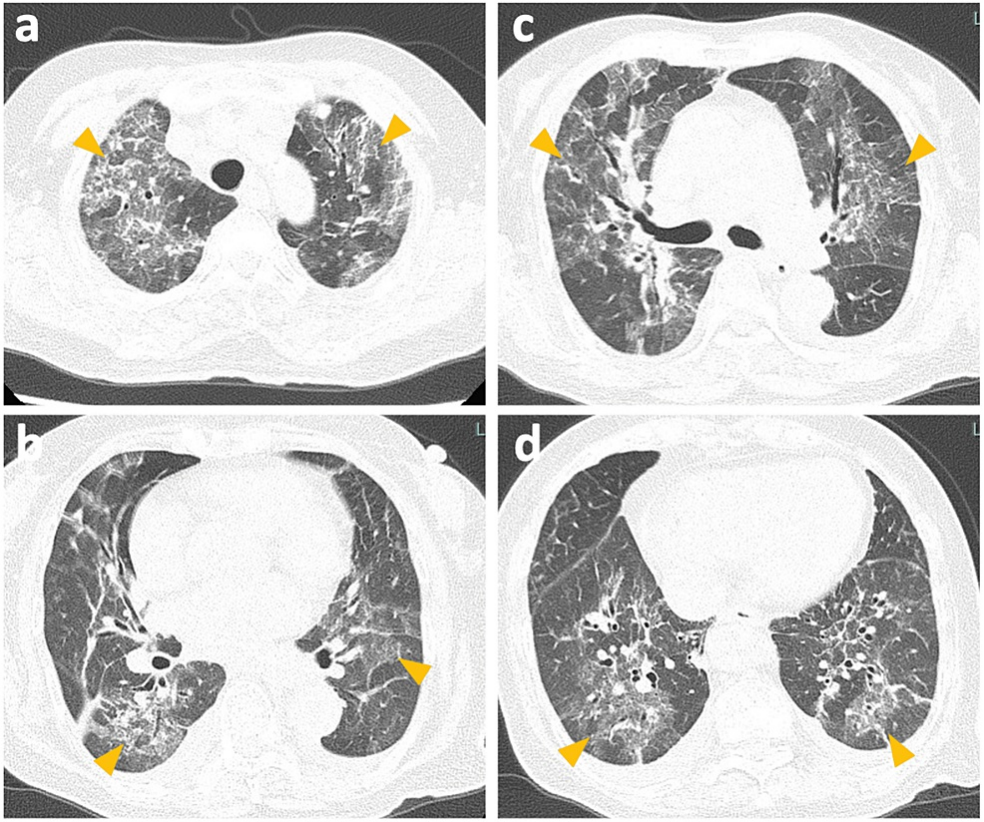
(a-d) Chest CT images of the patient on admission showing diffuse, unevenly distributed ground-glass opacities throughout both lungs (arrowheads)
